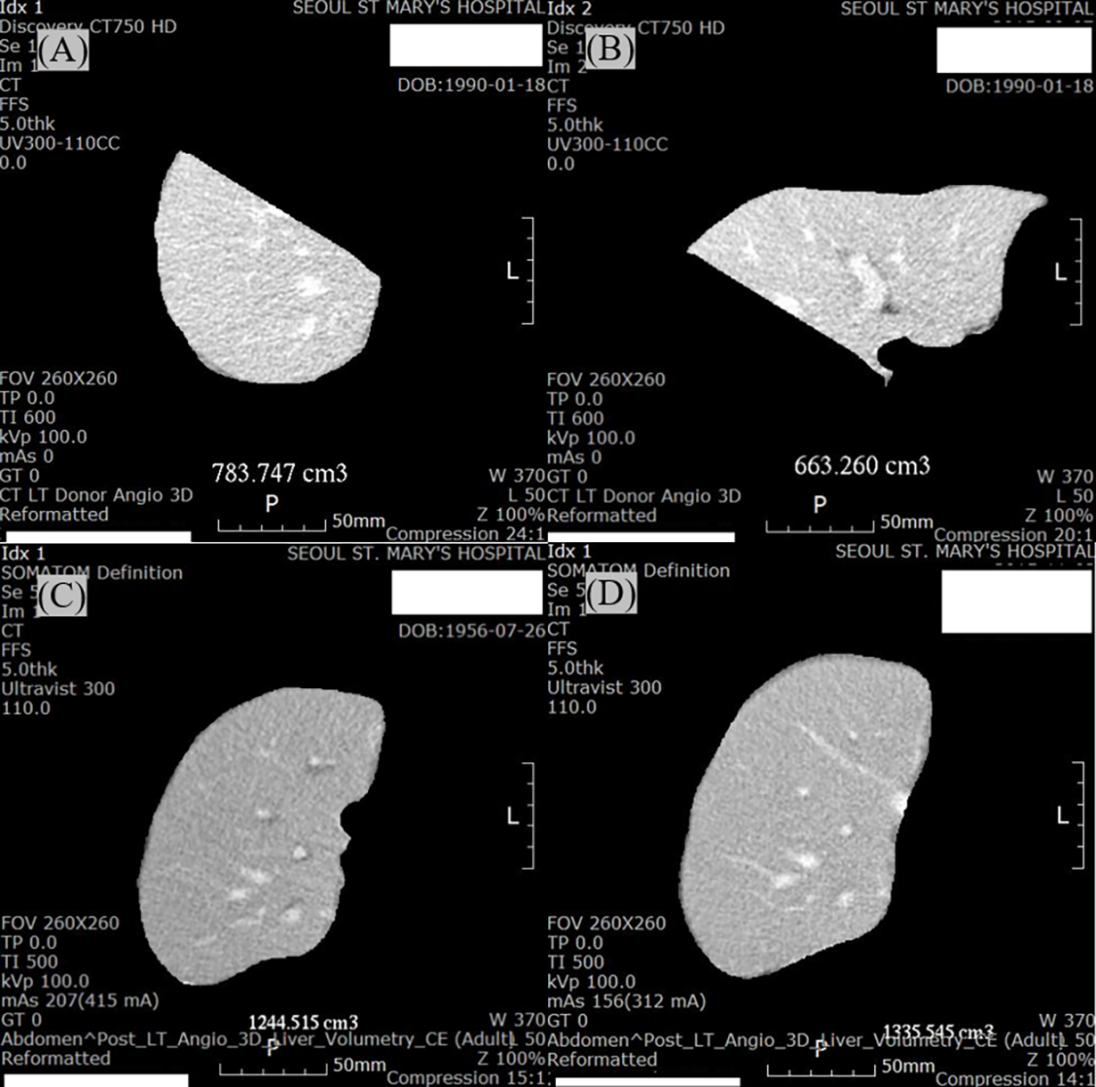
Volumetric computed tomography images in the right (A) and left (B) lobes of a donor preoperatively, and in the right lobe of a liver graft on postoperative days 7 (C) and 21 (D) in a patient who underwent a living donor liver transplantation.
